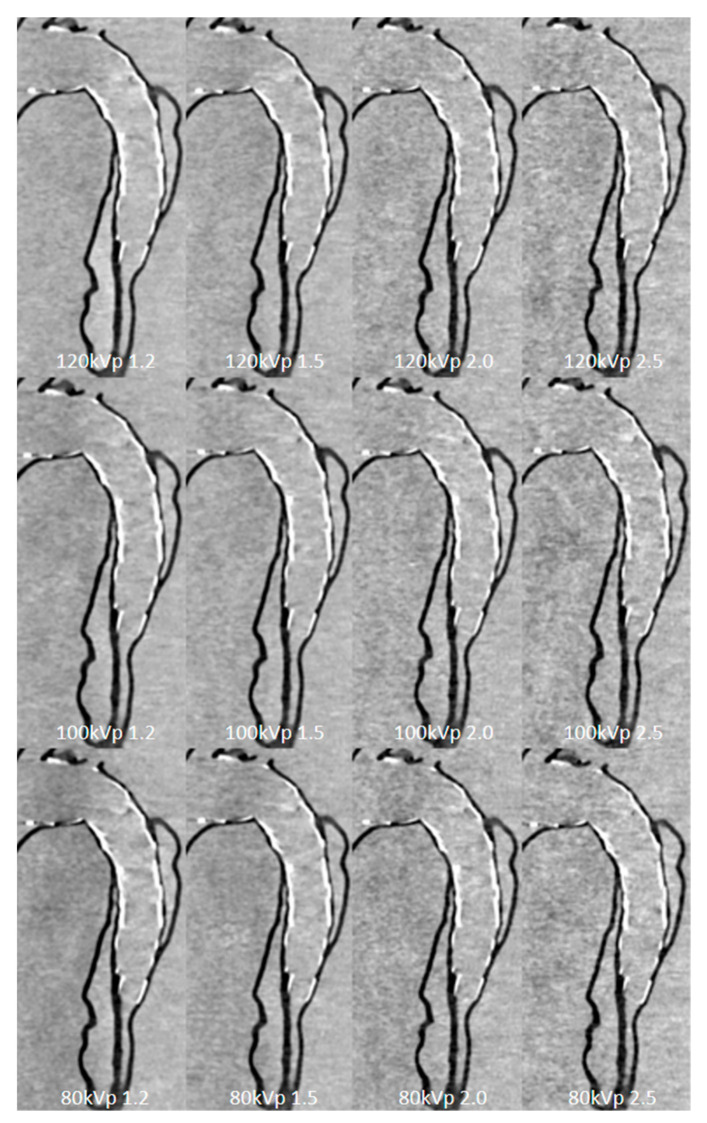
Sagittal reformatted images of CTA protocols. When kVp was decreased to 80, image noise increased with the use of high-pitch protocol values of 2.0 and 2.5. CTA: computed tomography angiography; kVp: kilovoltage peak. Reprinted with permission under open access from Wu et al. [113].
